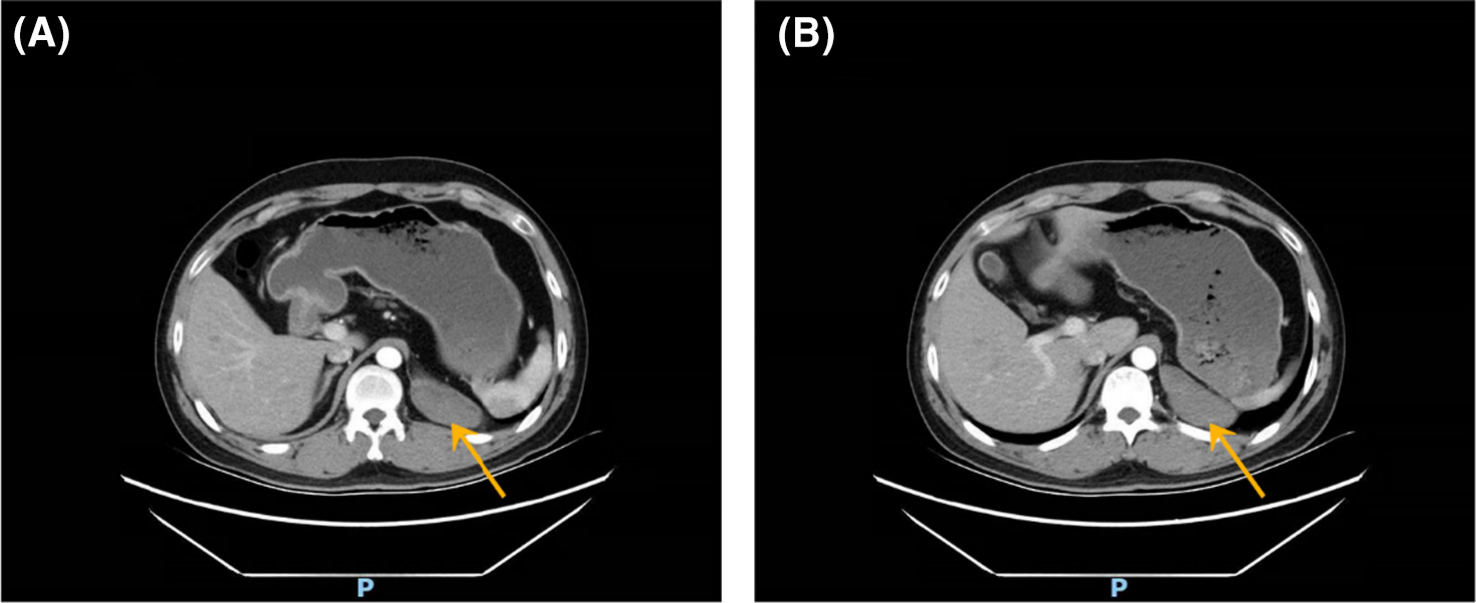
Computed tomography scan of the abdomen showing a well‐delineated soft tissue structure measuring 7.5 × 2.5 cm in the left diaphragm area, (A and B) are both arterial phase images, showing the mass at different levels, which may indicate the suspected blood supply artery from the abdominal aorta. An orange arrow has been used to mark the location of the mass.
